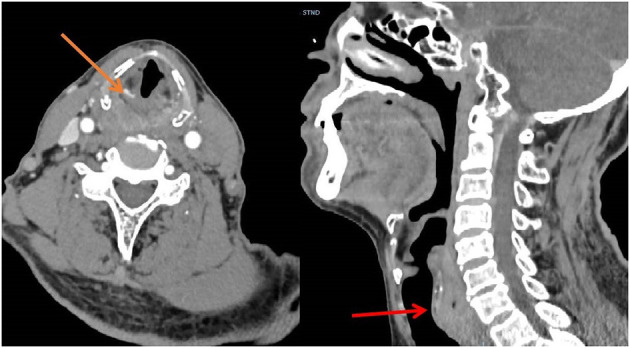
CT showed upper esophageal cancer with diffuse thickening and calcification.
